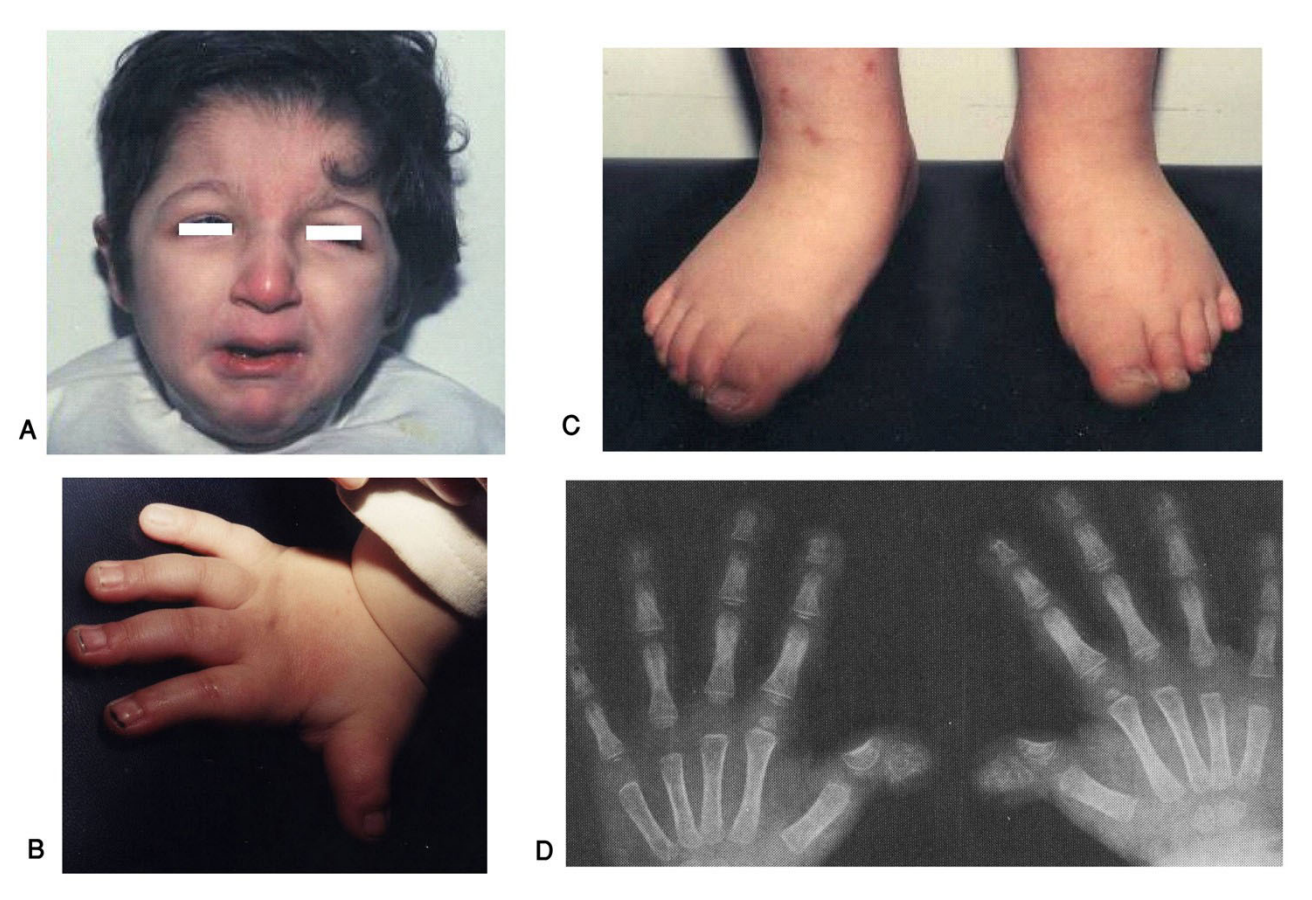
An Egyptian patient with Rubinstein-Taybi syndrome. Facial features (A), left hand and feet showing broad thumb and big toes (B, C) and X-ray of both hands showing short broad thumbs (D). (Limb Malformations & Skeletal Dysplasia Clinic, MSU, NRC).
